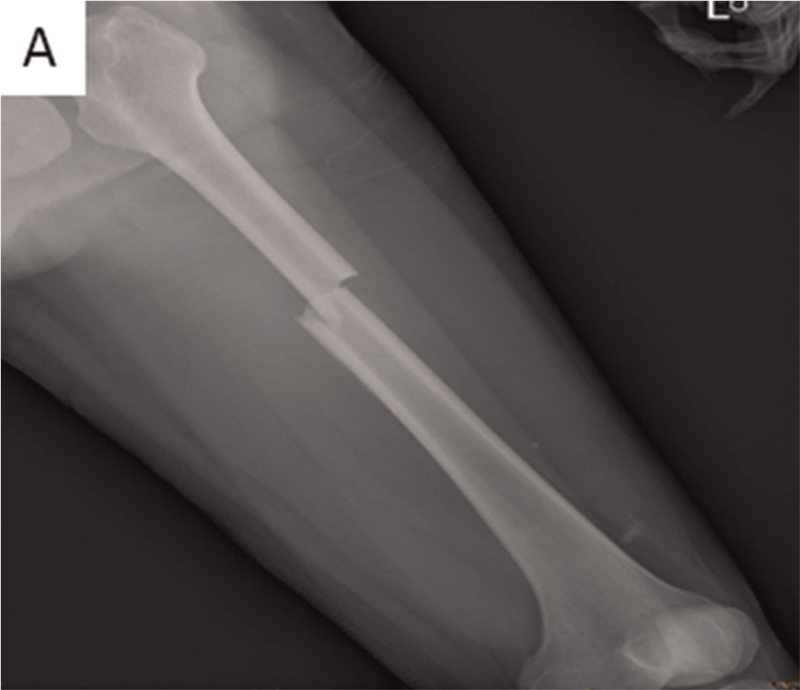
Radiograph showing left femoral shaft fracture.
